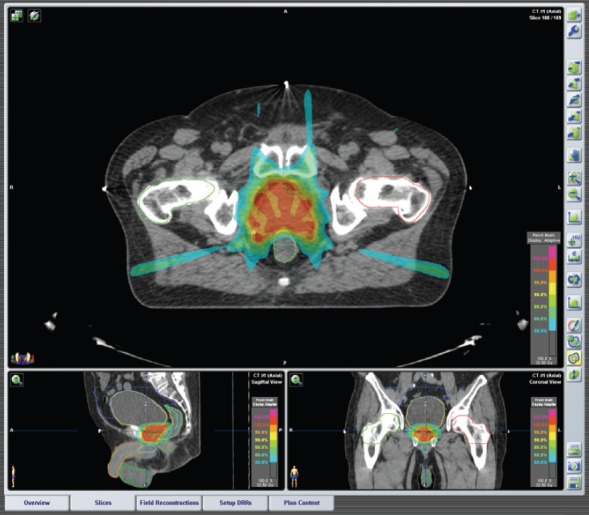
Plan of IMRT Prostate cancer, stage: cT1c cN0 cM0. IMRT treatment plan: Seven fixed beams, Energy: 6MV XRays. Total dose: 32.5 Gy in five fractions (6.5 Gy/fraction) every other day.
